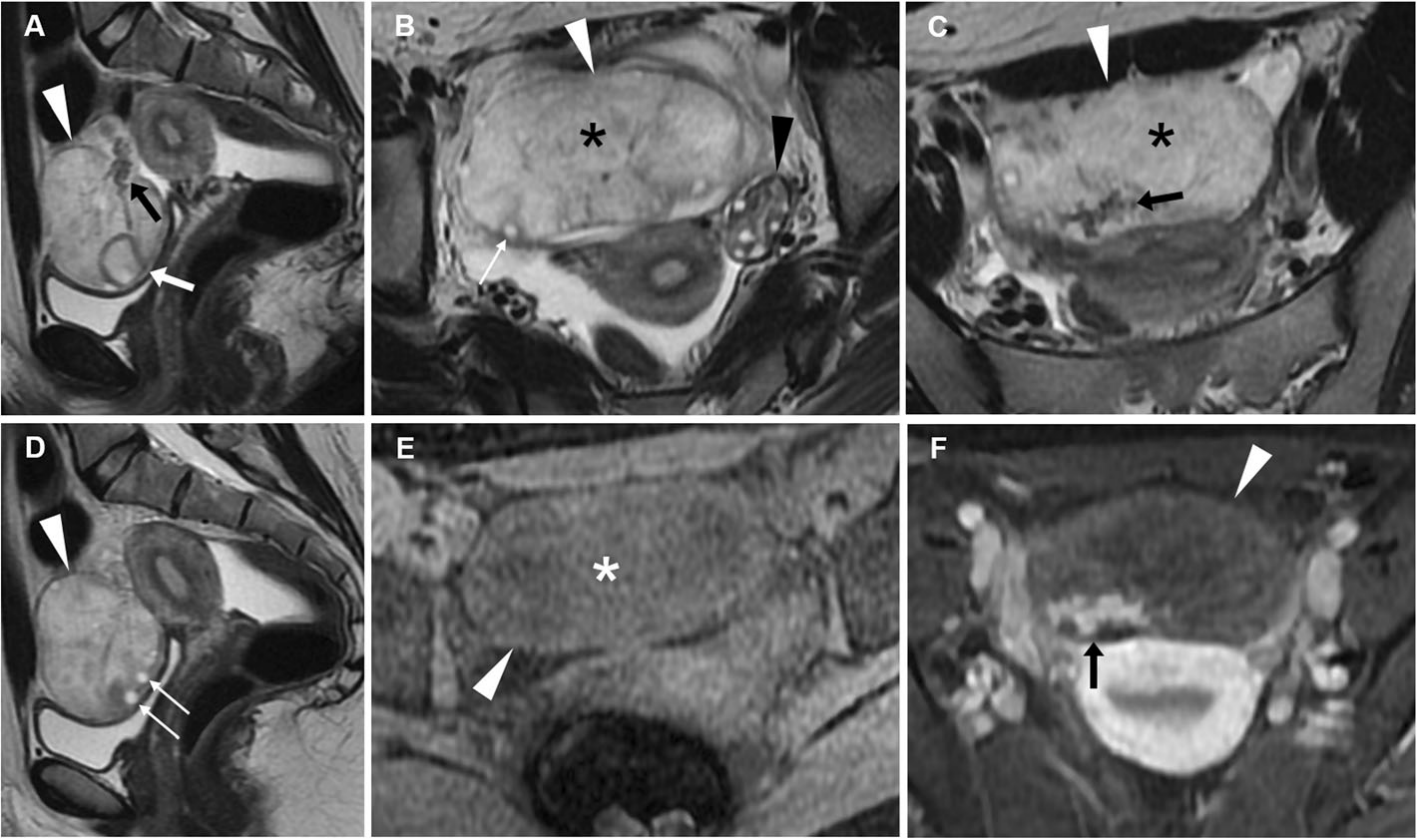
Right adnexal torsion in a 24-year-old woman with acute abdominal pain and leukocytosis. Multiplanar T2-weighted (a–d), oblique-axial precontrast fat-suppressed T1-weighted (e) and oblique-axial gadolinium-enhanced fat-suppressed T1-weighted (f) images show an enlarged (10 cm diameter) right ovary (arrowheads) with afollicular T2-hyperintense central stroma (asterisk in b and c), peripheral follicles (‘pearl string sign’, thin arrows in b and d) and corpus luteum (arrow in a). The normal left ovary is also seen (black arrowhead in b). Note the engorged blood vessels along the posterior portion of the right adnexa (black arrows in a, c and f). The enlarged right ovary shows homogeneous intermediate T1-weighted signal intensity (asterisk in e), without hyperintense haemorrhagic changes, reflecting the early stage of torsion. The patient underwent laparoscopic detorsion with adnexal sparing
